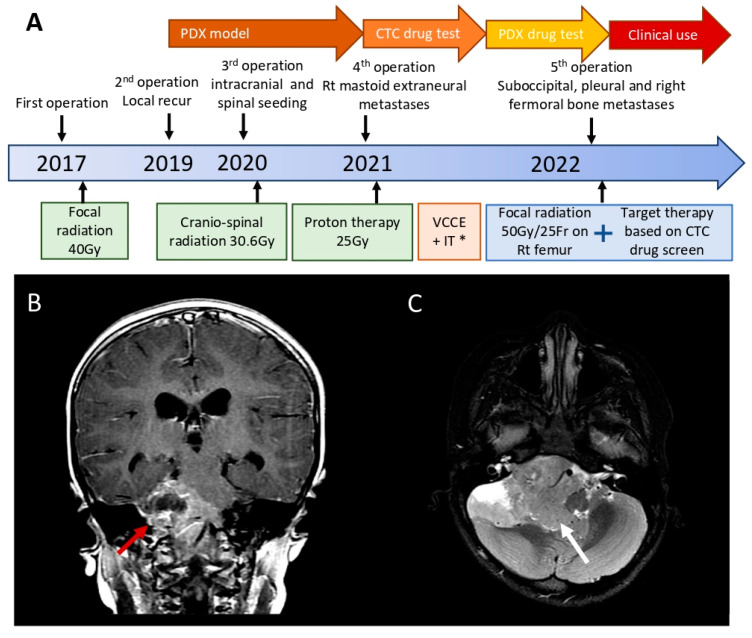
Primary ependymoma on the right cerebello-pontine angle with brain stem compression and extraneural metastasis in a 6-year-old girl. (A) Timeline of disease course and recurrence. * VCCE: vincristine, cisplatin, cyclophosphamide, etoposide; IT: intrathecal chemotherapy. (B) T1-weighted MR image with contrast enhancement showing the initial tumor mass wrapping the lower cranial nerve on the right cerebello-pontine angle (red arrow). (C) Axial view of the diffusion-weight image showing the iso-dense tumor mass compressing the brain stem (white arrow).
